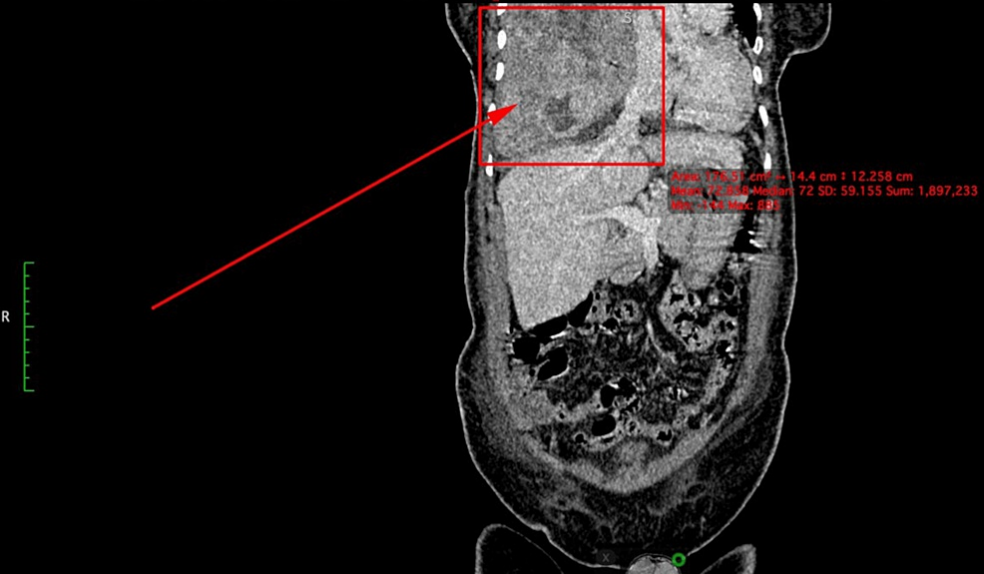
CT coronal IV contrast image demonstrates caudal effacement of the diaphragm with mass effect on the subdiaphragmatic structures such as the liver. CT: computed tomography; IV: intravenous
